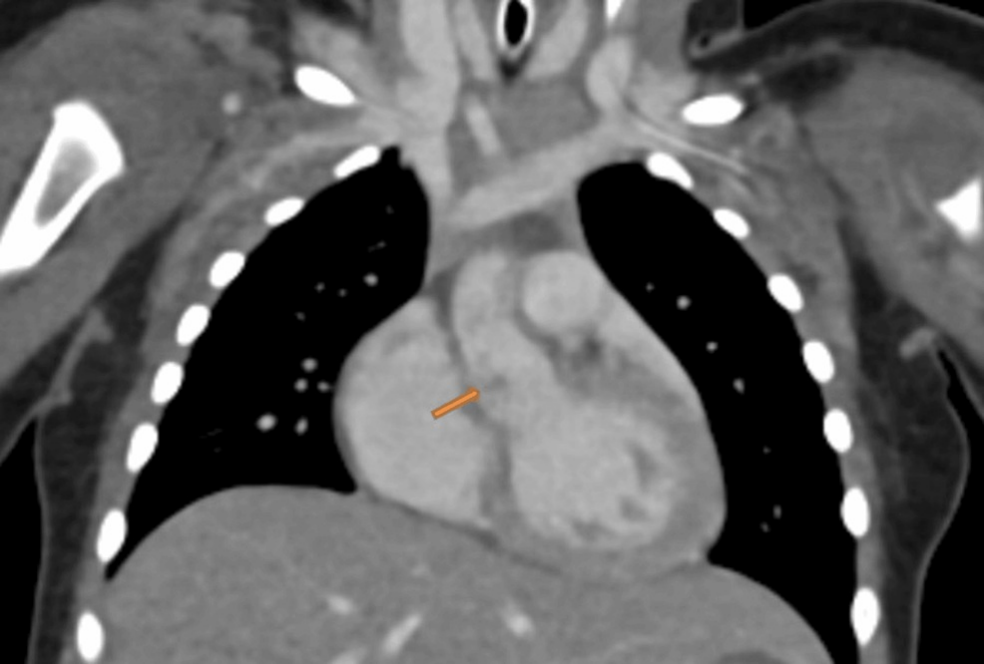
Contrast-enhanced thoracic computed tomography angiography shows evidence of aortic root dilatation with the presence of an ill-defined hypodense filling defect (arrow, presumed vegetation).
